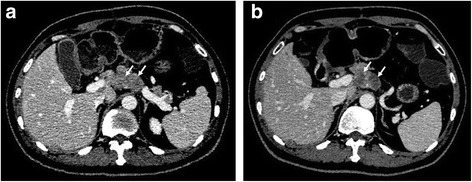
A pancreatic neuroendocrine tumor (G2) with retroperitoneal lymph node metastases in one’s fifties. The pre-treatment CT scan showed (a) retroperitoneal fusion nodules with a relatively low density (white arrows, size: 2.0 cm, density: 82 HU) in front of the abdominal artery. (b) The nodules exhibited a slight reduction in size and an obvious reduction in density (white arrows, size: 1.9 cm, density: 56 HU) at the first evaluation after treatment with sunitinib. The percent change in tumor size and density was 5.0% and 31.7%, respectively. This patient was classified as PR according to the Choi criteria but as SD based on RECIST
